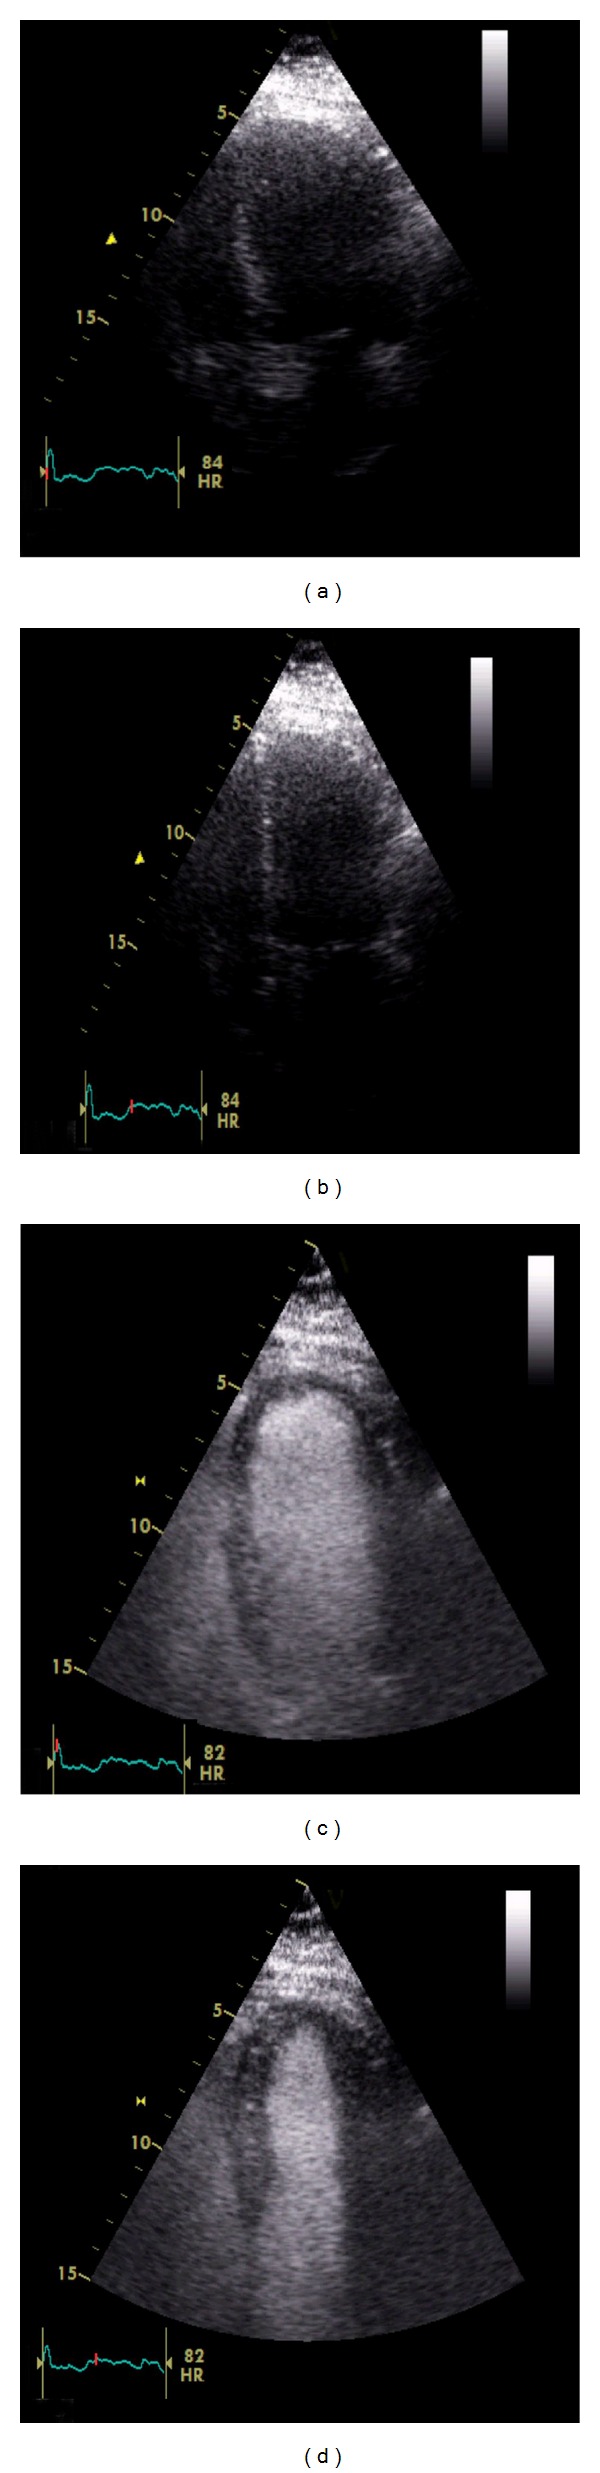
Apical 4-chamber images taken before ((a) and (b)) and after ((c) and (d)) ultrasound contrast injection in a patient attending for routine transthoracic echocardiography. At rest, endocardial definition at end diastole (a) and end systole (b) was poor and did not permit quantitation of ejection fraction or assessment of regional (segmental) function. Following a single bolus injection of contrast, endocardial border visualisation was significantly improved at end diastole (c) and end systole (d), allowing regional and global systolic assessments.
